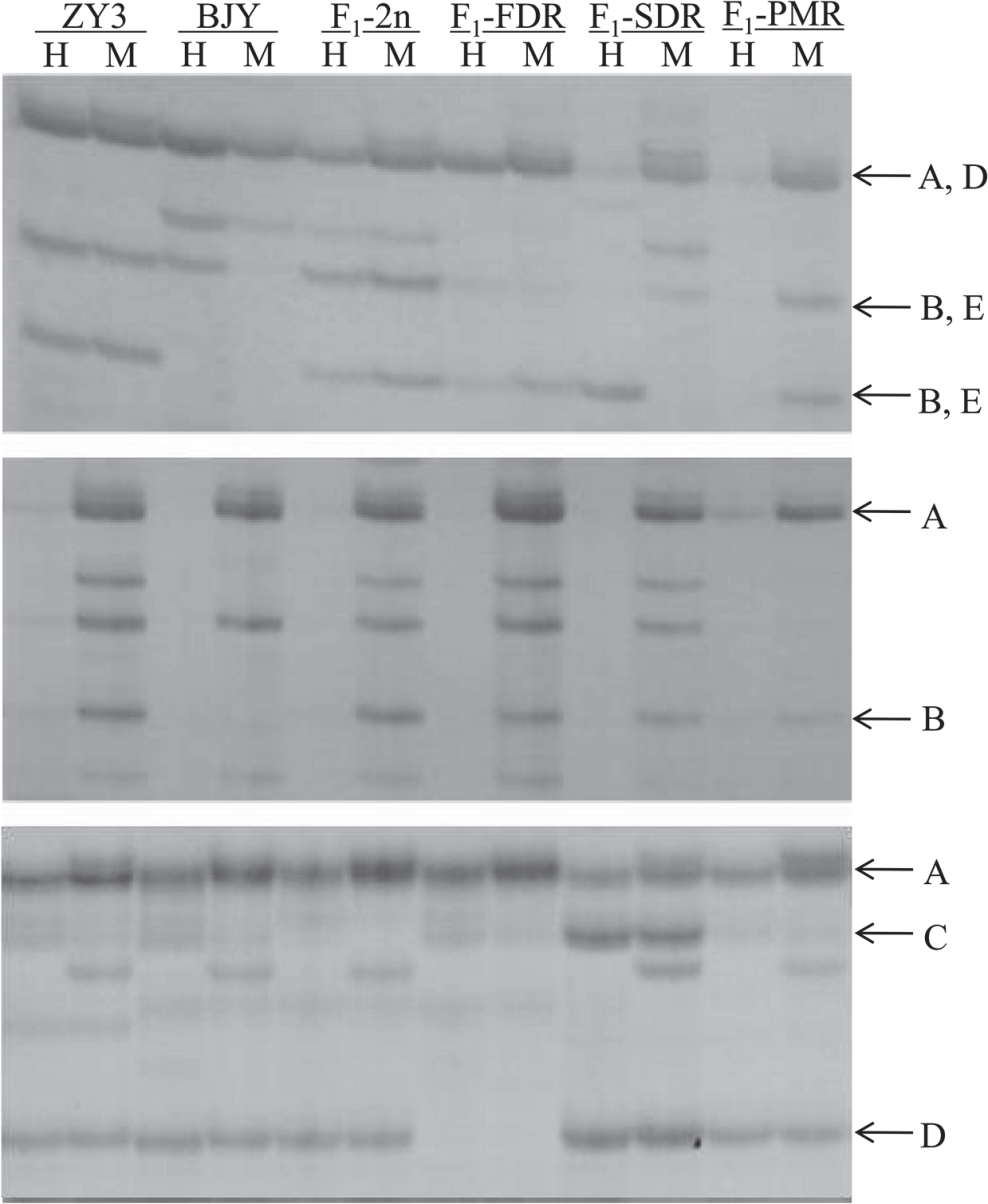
Examples of MSAP profiles showing the inheritance and variation of DNA methylation sites in F1 hybrids relative to their parental lines. H genomic DNA was digested with *Hpa*II and *Eco*RI; M genomic DNA was digested with *Msp*I and *Eco*RI; Primer combinations are E_00_+GC/ HM_00_+CT (a), E_00_+CC/HM_00_+TC (b), E_00_+GG/ HM_00_+TG (c). (A) Monomorphic inheritance; (B) Additivity inheritance; (C) Demethylated; (D) Hyper- methylated; (E) Unidentified types.
